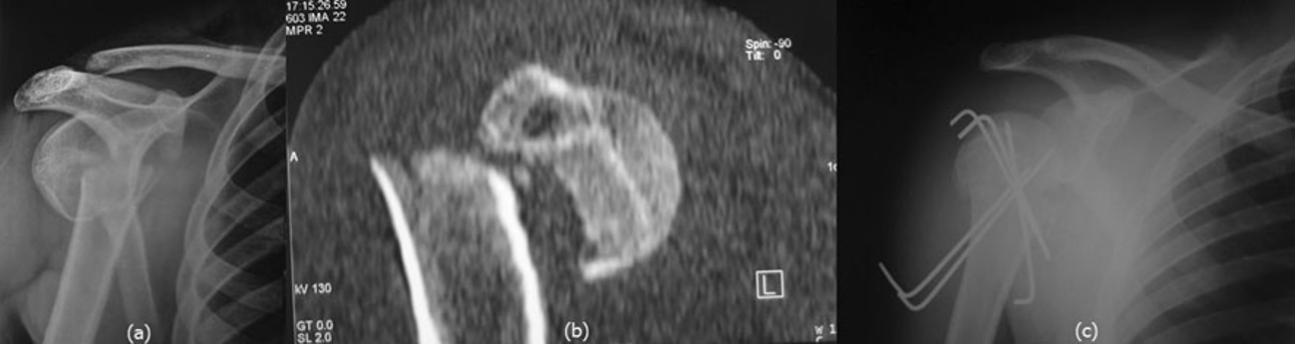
a Anteroposterior radiograph showing fracture of surgical neck of humerus. b CT scan image showing the fracture pattern with angulation. c Postoperative reduction with multiple K wires
